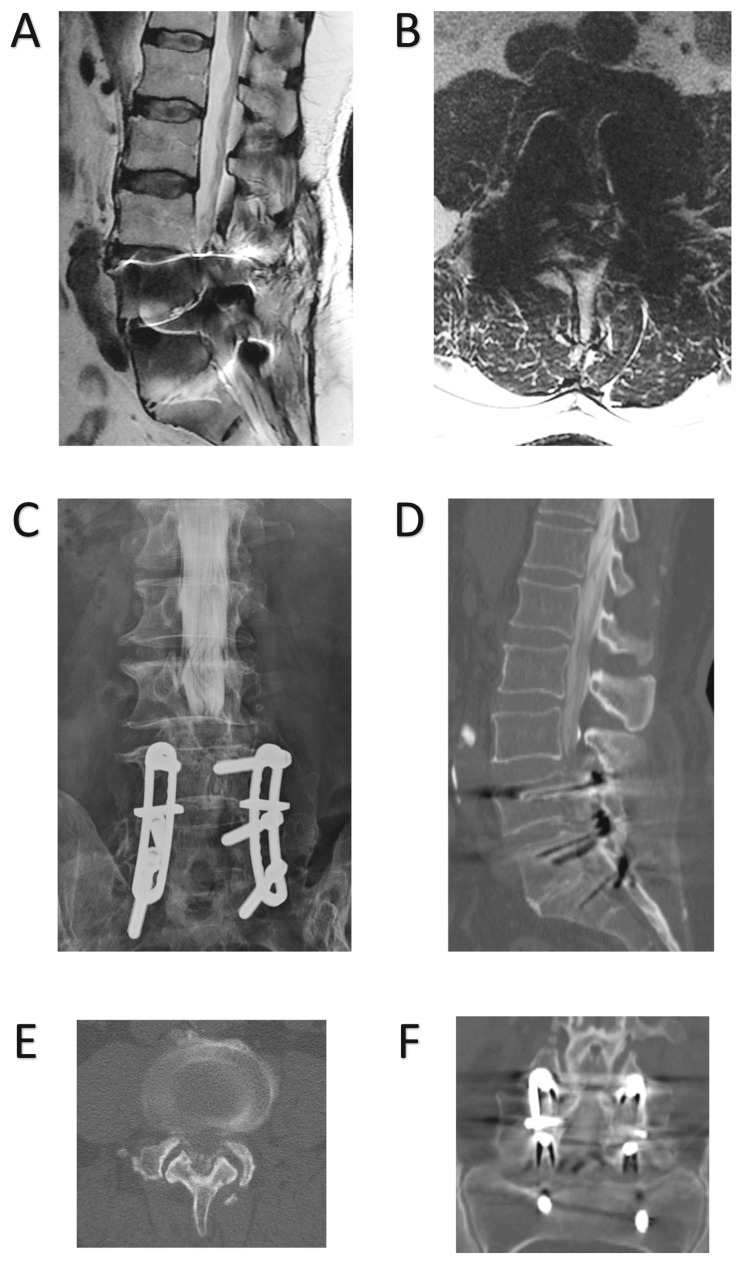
Advanced imaging findings on presentation (A-B) Mid-sagittal T2-weighted MRI (A) as well as a corresponding axial cut through the L3-4 disc space (B) demonstrated adjacent segment degeneration with L3-4 canal stenosis. (C-E) Myelogram (C), mid-sagittal (D), and L3-4 axial (E) cuts from a subsequent CT with similar findings. (F) CT myelogram also confirmed successful L4-S1 posterolateral fusion, which can be appreciated in this coronal image MRI: magnetic resonance imaging, CT: computed tomography
